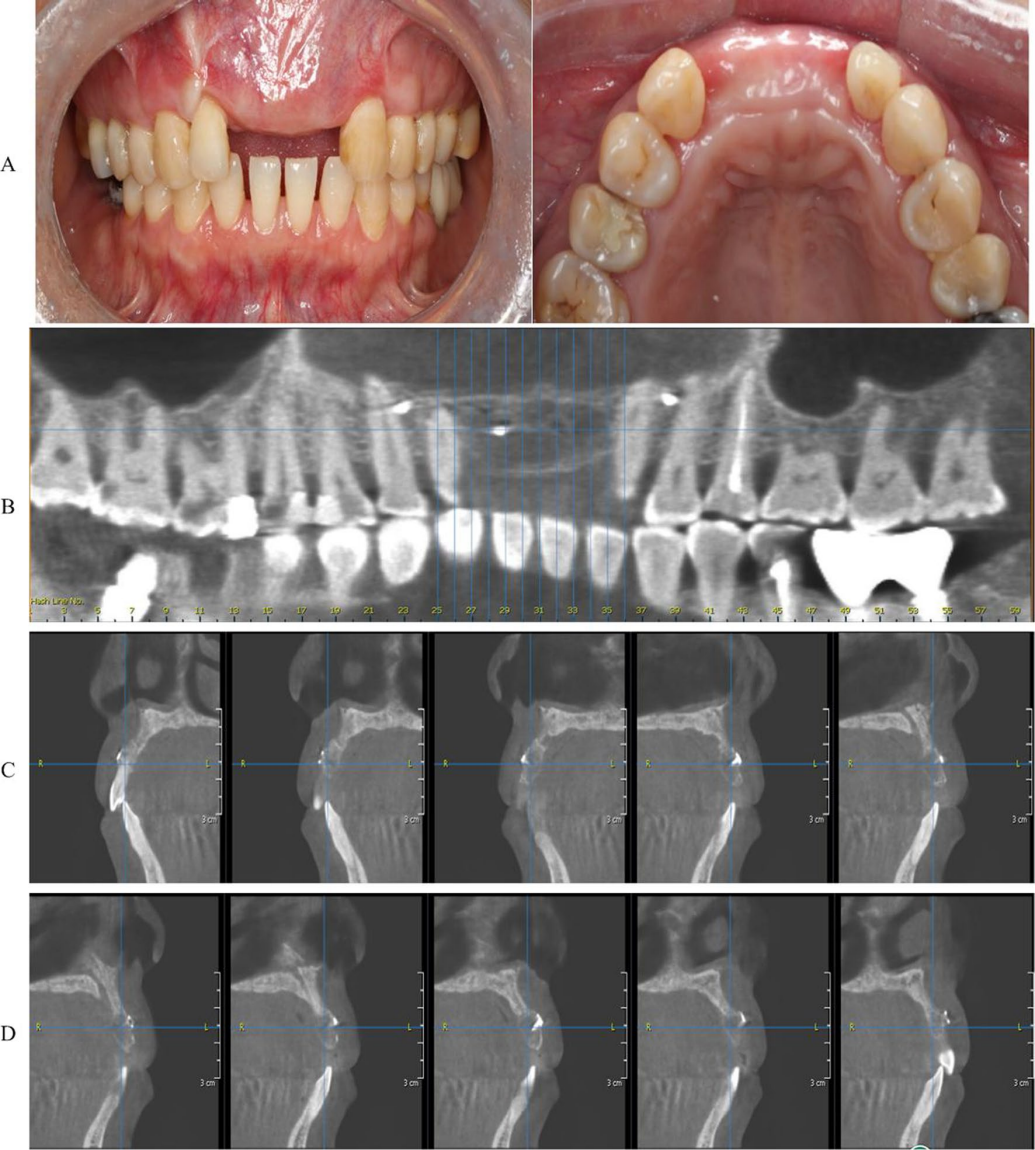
Healing and bone resorption revealed by cone beam CT at 4 months postoperatively: A intraoral photo; B bone graft position; C, D cone beam CT shows local bone resorption
